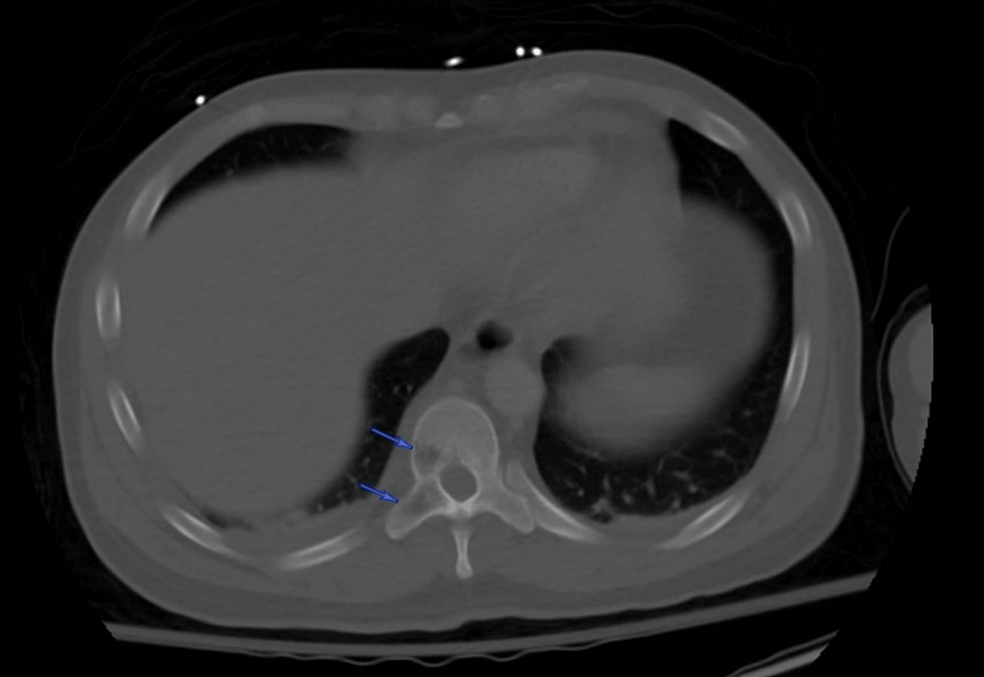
Axial bone window CT scan of the chest with intravenous contrast revealing lytic T10 vertebral lesions (blue arrows) CT: computed tomography
